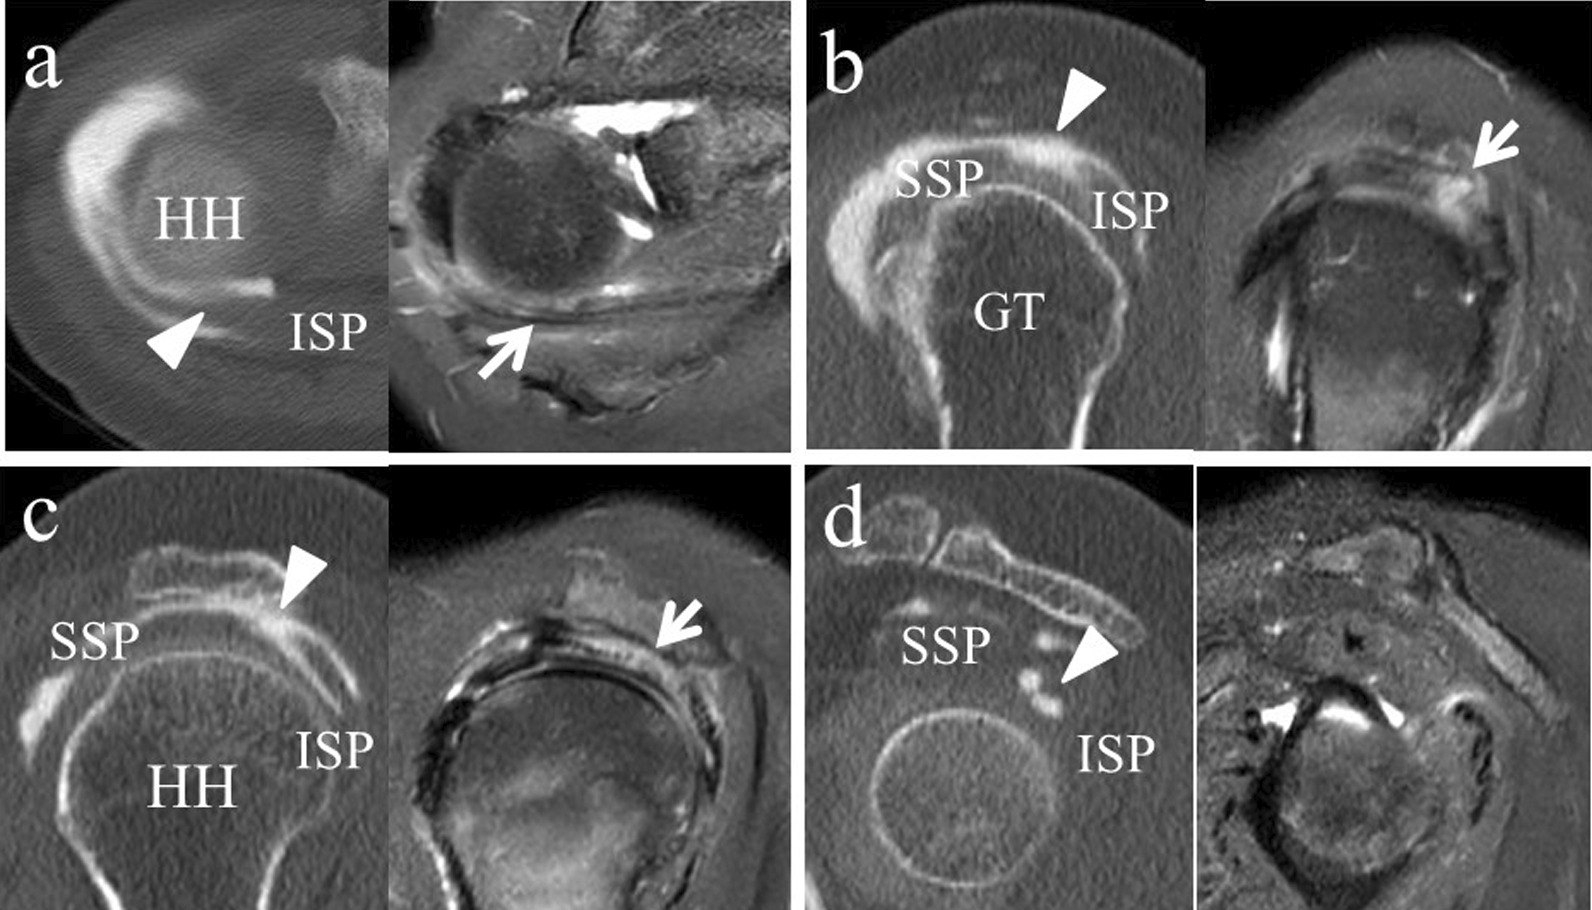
The tear is clearly visualized by Computed tomography bursography. On fat-suppressed T2-weighted Magnetic resonance imaging, there appears to be increased signal in the infraspinatus, suggesting a bursal-side tear. a Axial image shows longitudinal pooling of contrast medium in the infraspinatus (arrowhead) on Computed tomography bursography. The Magnetic resonance imaging shows areas of an increased signal in the infraspinatus (arrows). On Computed tomography bursography (b, c), the oblique sagittal image reveals that the tear communicates with the subacromial bursa in the infraspinatus tendon (arrowhead). The Magnetic resonance imaging shows areas of increased signal in the infraspinatus tendon, which is in contact with the bursal side of the rotator cuff (arrows). d Computed tomography bursography shows pooling of contrast medium within the muscle (arrowhead). *HH* head of the humerus, *GT* greater tubercle, *SSP* supraspinatus, *ISP* infraspinatus
